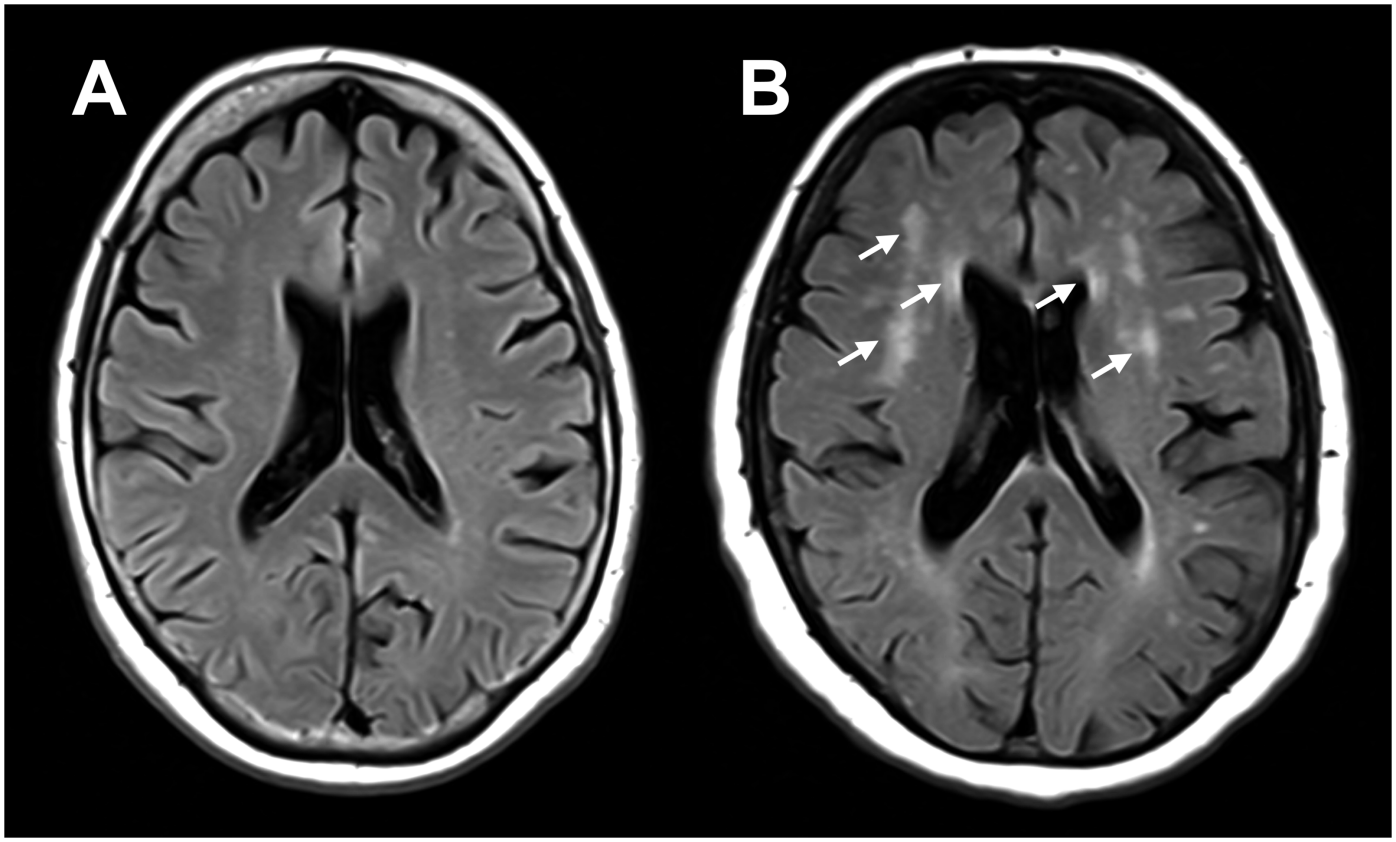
FLAIR images of two different representative subjects with different WMH load. Subject A represents lower WMH load (i.e. a WMH volume ≤ 0.5% of the intracranial volume that corresponded approximately to Fazekas grade 0–1) and subject B higher WMH load (i.e. a WMH volume ≥ 1% of the intracranial volume that corresponded approximately to Fazekas grade 2–3), with arrows indicating example regions with WMH.
